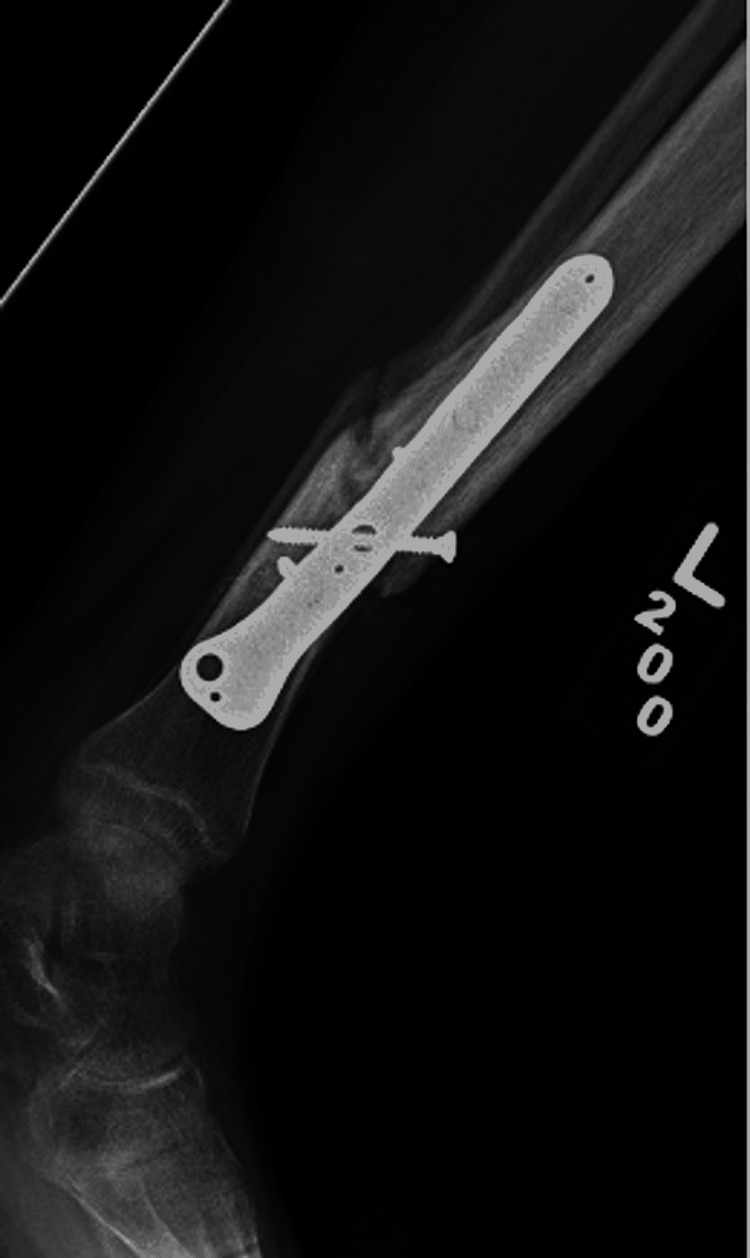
Post-operative lateral tibia-fibula radiographs of revision open reduction and internal fixation with removal of flexible nails of tibial shaft.
